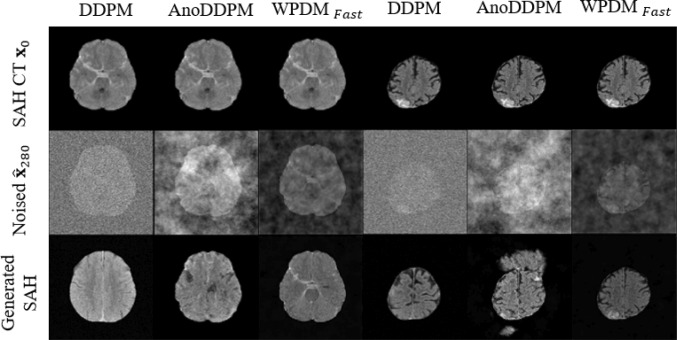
Comparison of generative model results. Two representative SAH CT images were selected to compare the outputs of DDPM (Gaussian noise), AnoDDPM (Simplex noise), and $$\hbox {WPDM}_{\text {Fast}}$$ (Worley-Perlin noise Fast)
